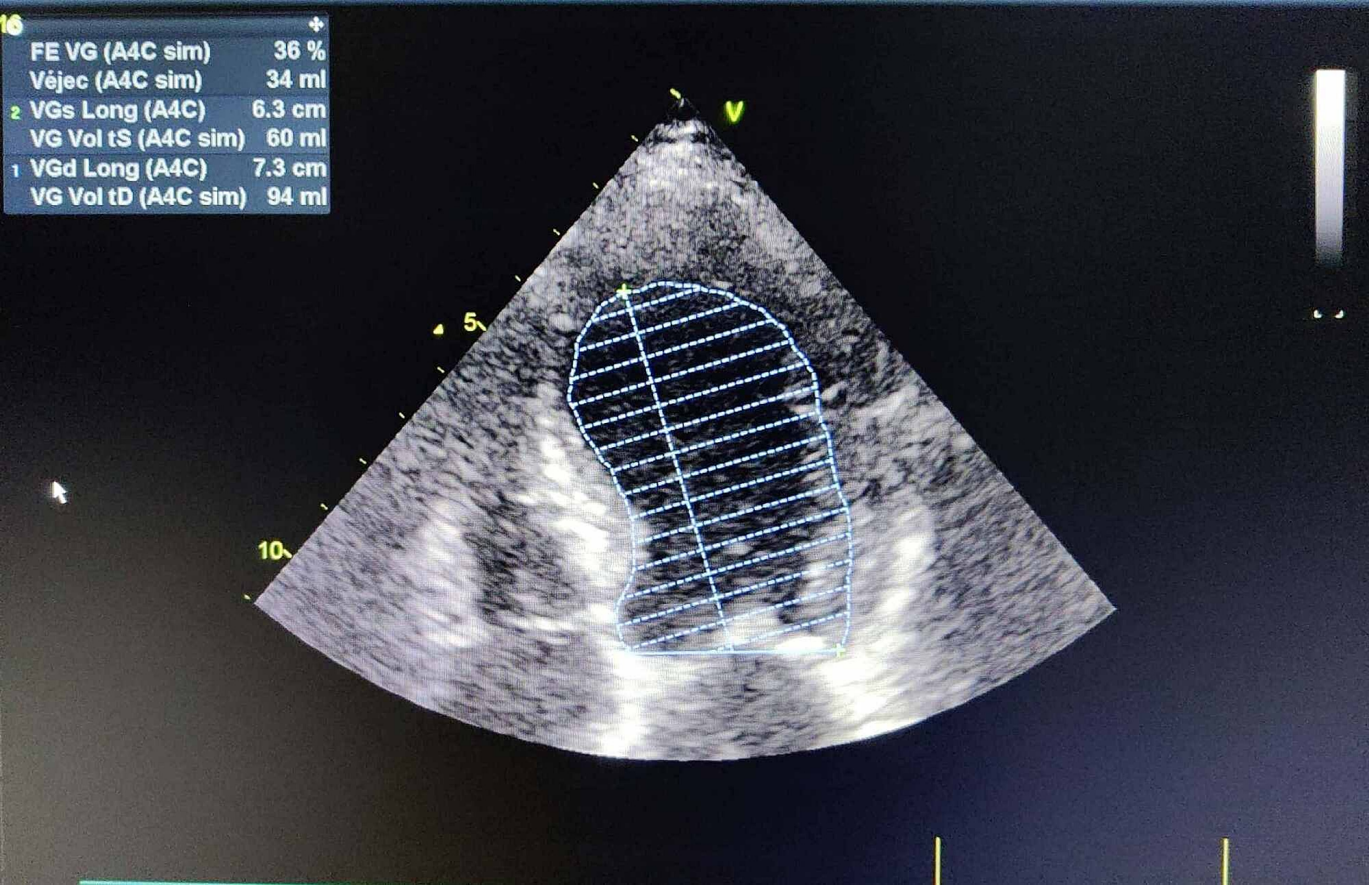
Echocardiography showed a severe VG dysfonction
